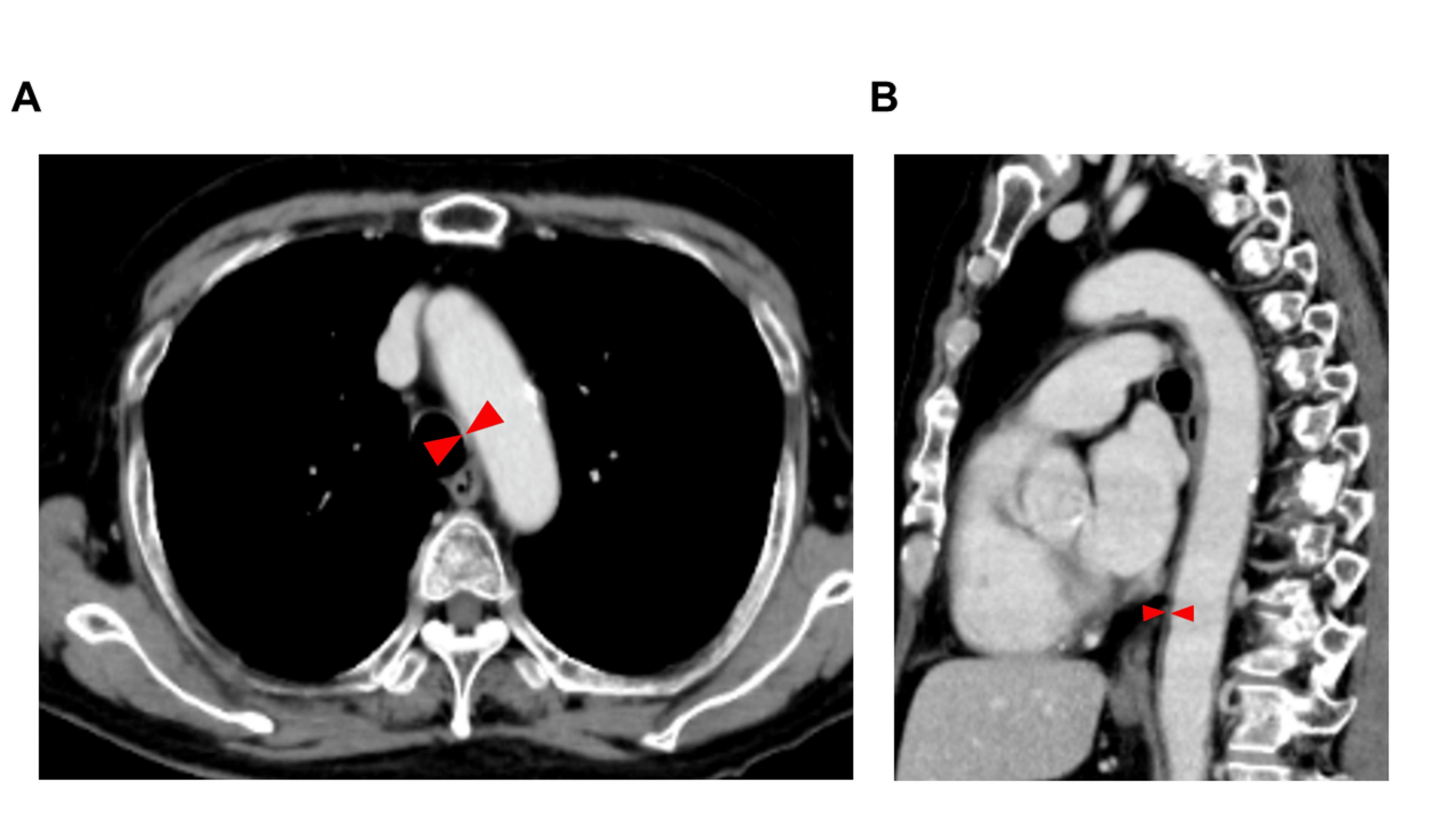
(A) Progression of findings on contrast-enhanced CT: a horizontal view. (B) Progression of findings on contrast-enhanced CT: a sagittal view. (A) A subsequent CT scan post-third docetaxel cycle (day 39 from initial docetaxel) demonstrated sustained reduction of aortic wall thickening. (B) This figure displays a sagittal view.  Red arrows are used to compare the aortic wall thickness.
